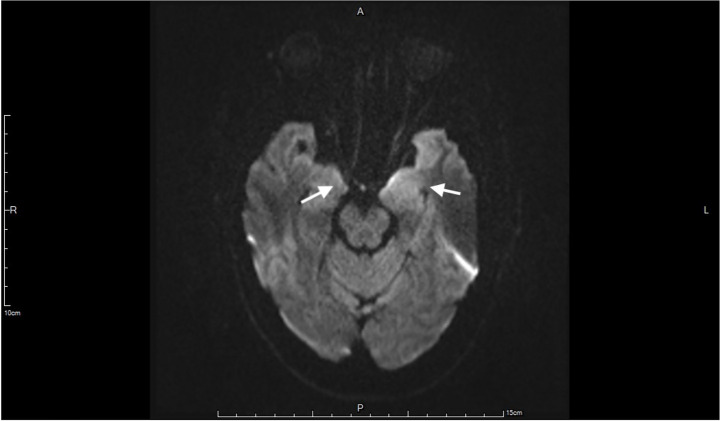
Bilateral hippocampus and amygdala are markedly swollen with high signal in DWI (white arrows) of patients with Anti-NMDA receptor encephalitis.
